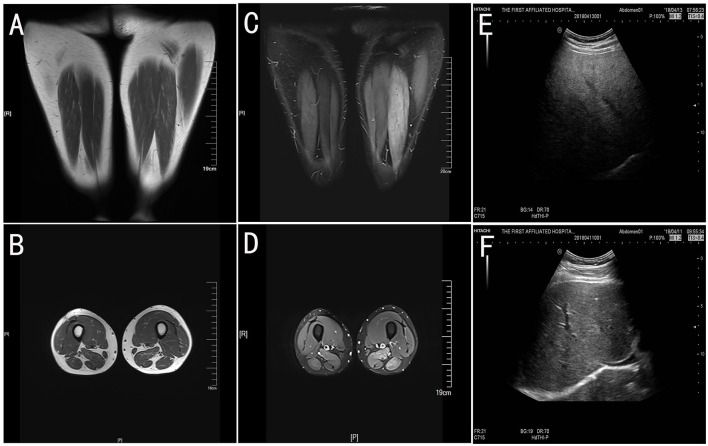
The muscle MRI of lower limbs and abdomen ultrasound. The T1-weight images (A, B) showed slightly atrophy of bilateral lower limbs. Fat suppression (T2-weighted short tau inversion recovery, T2-STIR) images (C, D) have high signal intensity in the bilateral limb muscles, which indicates muscle injury. The abdomen ultrasound (E) showed obvious signs of a fatty liver compared with the health control (F).
